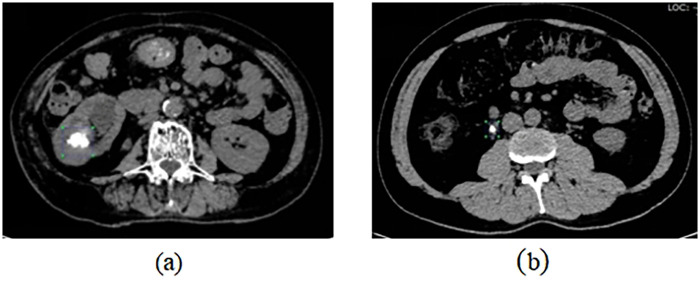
Annotation of stone regions in CT images using labelimg. (a) indicates kidney stone annotation, (b) indicates ureteral stone annotation.
